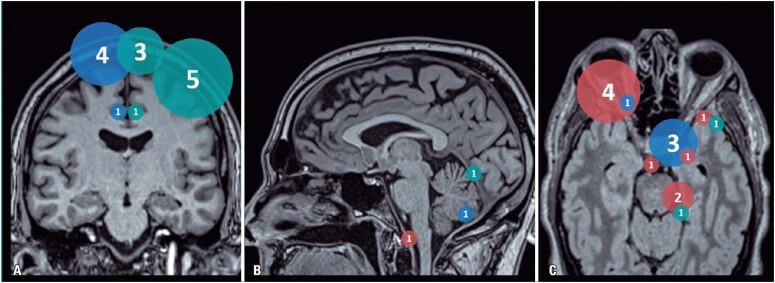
Location of the analyzed meningiomas. A normal MRI study in the coronal (A), sagittal (B) and axial (C) planes was used as a template
